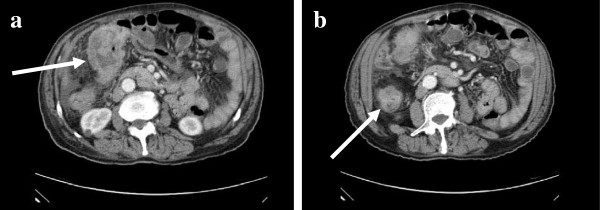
Abdominal computed tomography (CT) suggested a large tumor of hepatic flexure of the colon (a) (arrow). Another tumor of the cecum is visible on a different cut (b) (arrow).
